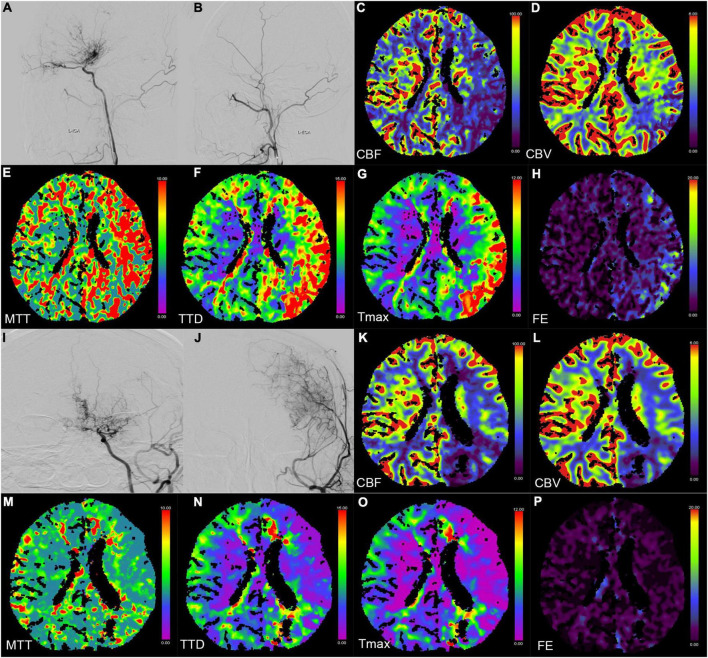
Digital subtraction angiography (DSA) and CT perfusion (CTP) images of a 23-year-old female patient with a history of headache for 2 months (A–H preoperation, I–P postoperation). (A,B,I,J) The DSA images show a patient bypass with supply to the majority of the middle cerebral artery territory after direct bypass surgery. (C–H,K–P) The CTP images show that the hemodynamics improved after revascularization in the left hemisphere.
